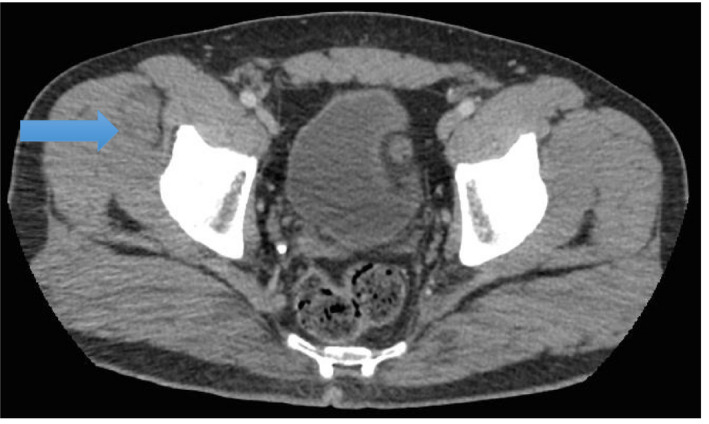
CT Abdomen/Pelvis showing soft tissue mass involvement at the level of the abdomen
